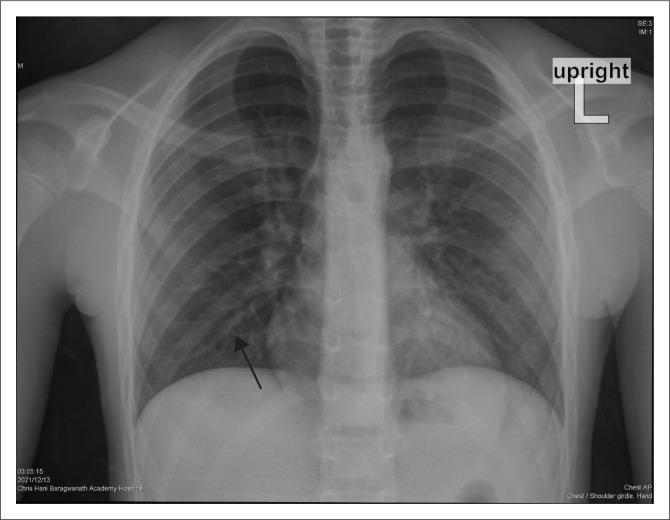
Frontal chest radiograph showing peribronchial thickening of the right lower lobe bronchus (black arrow) in a 9-year-old male patient with no comorbidities. The peribronchial thickening is seen bilaterally, implying widespread inflammation of the airways.
